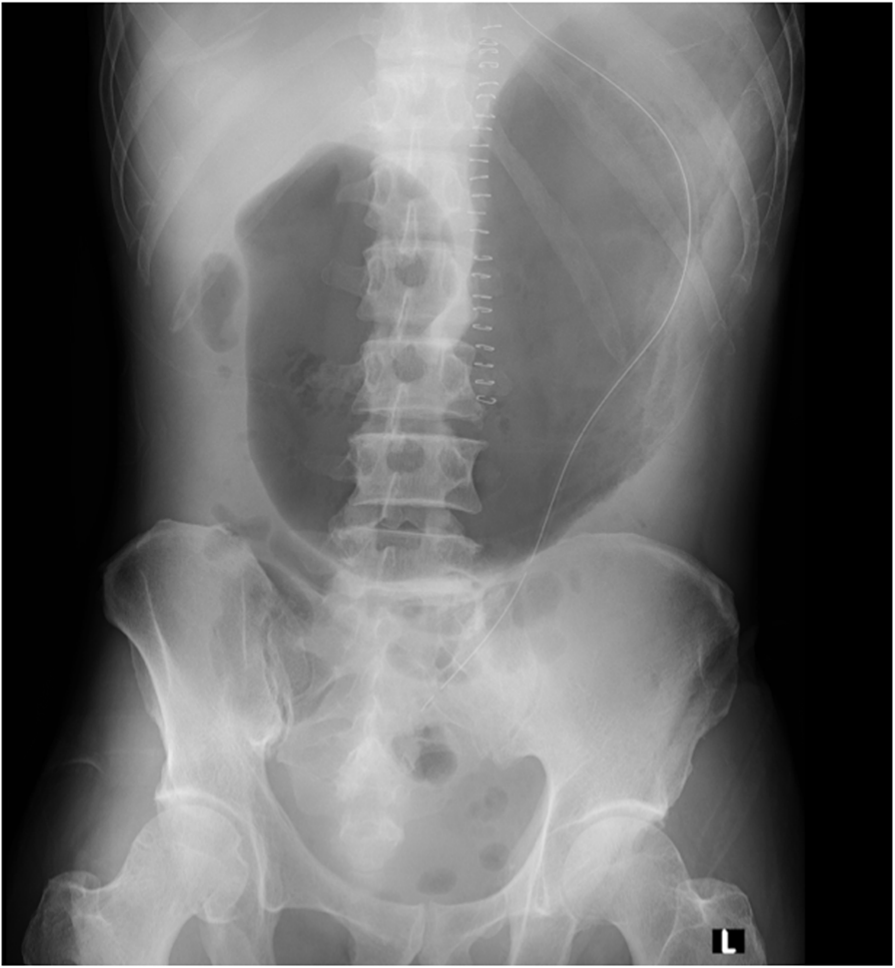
Abdominal radiograph on POD 10 showing the patient’s distended stomach, which was likely owing to aerophagia and nasogastric tube malfunction. The tube was repositioned to drain the air. POD, postoperative day
